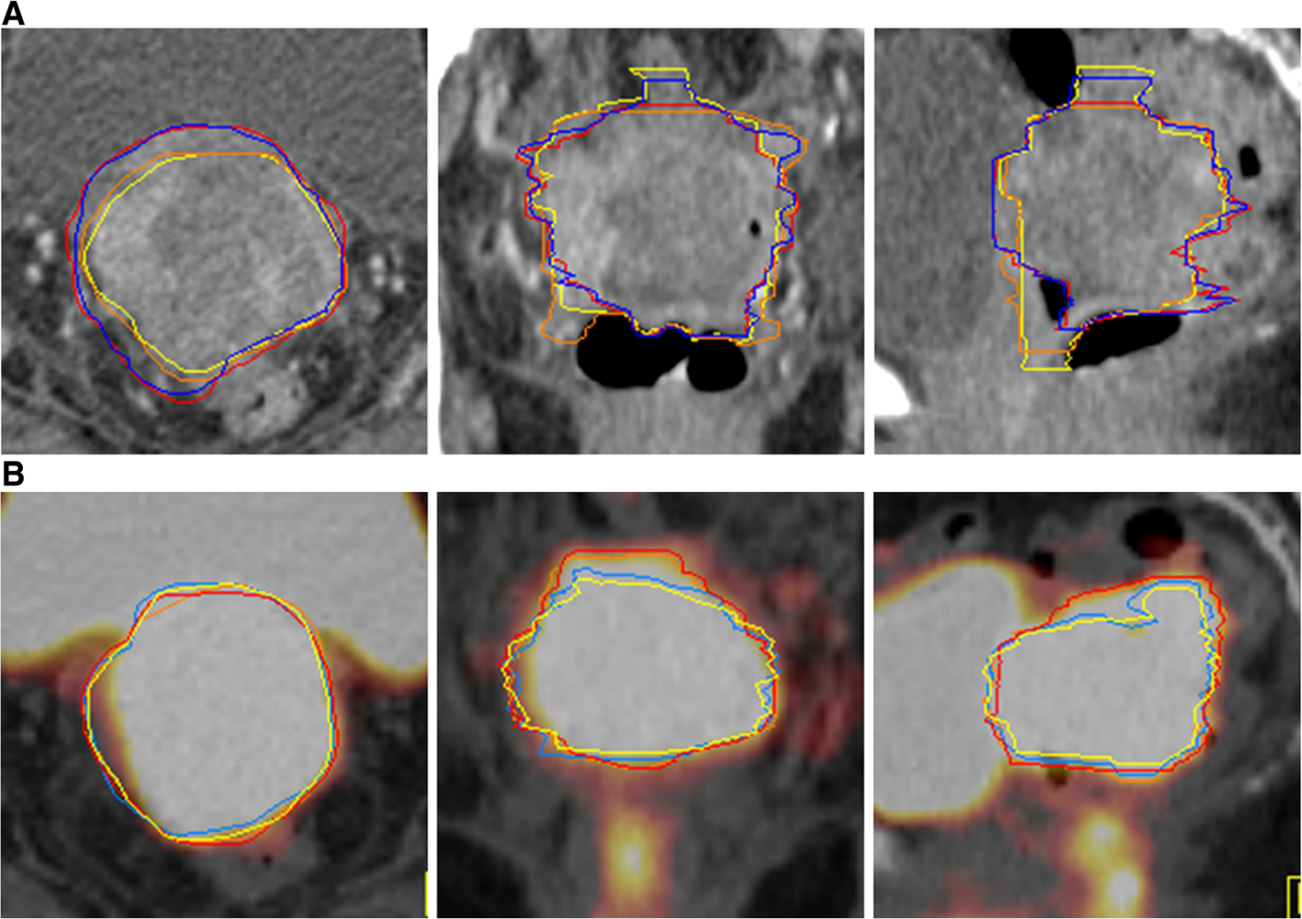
^18^F-FDG PET with coregistered contrast enhanced CT showed a local recurrence with a SUV_max _of 16.16 in a 60 year old patient 6 months after total hysterectomy, adnexectomy and pelvic lymphadenectomy for an endometrial cancer FIGO IIIA. (A) GTV_CTs_ defined by four observers on axial, sagittal and coronal contrast enhanced CT. (B) GTV_PET/CTs_ defined by four observers on axial, sagittal and coronal ^18^F-FDG PET/CT. In panel B we observed a greater interobserver agreement and the GTV_PET/CTs_ were smaller than the GTV_CTs_.
